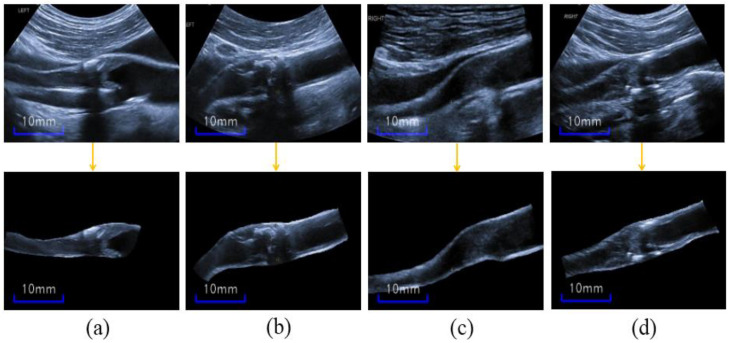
(a–d) Original ultrasound images (the first row) and the corresponding cropped-artery-blood-vessel images (the second row).
